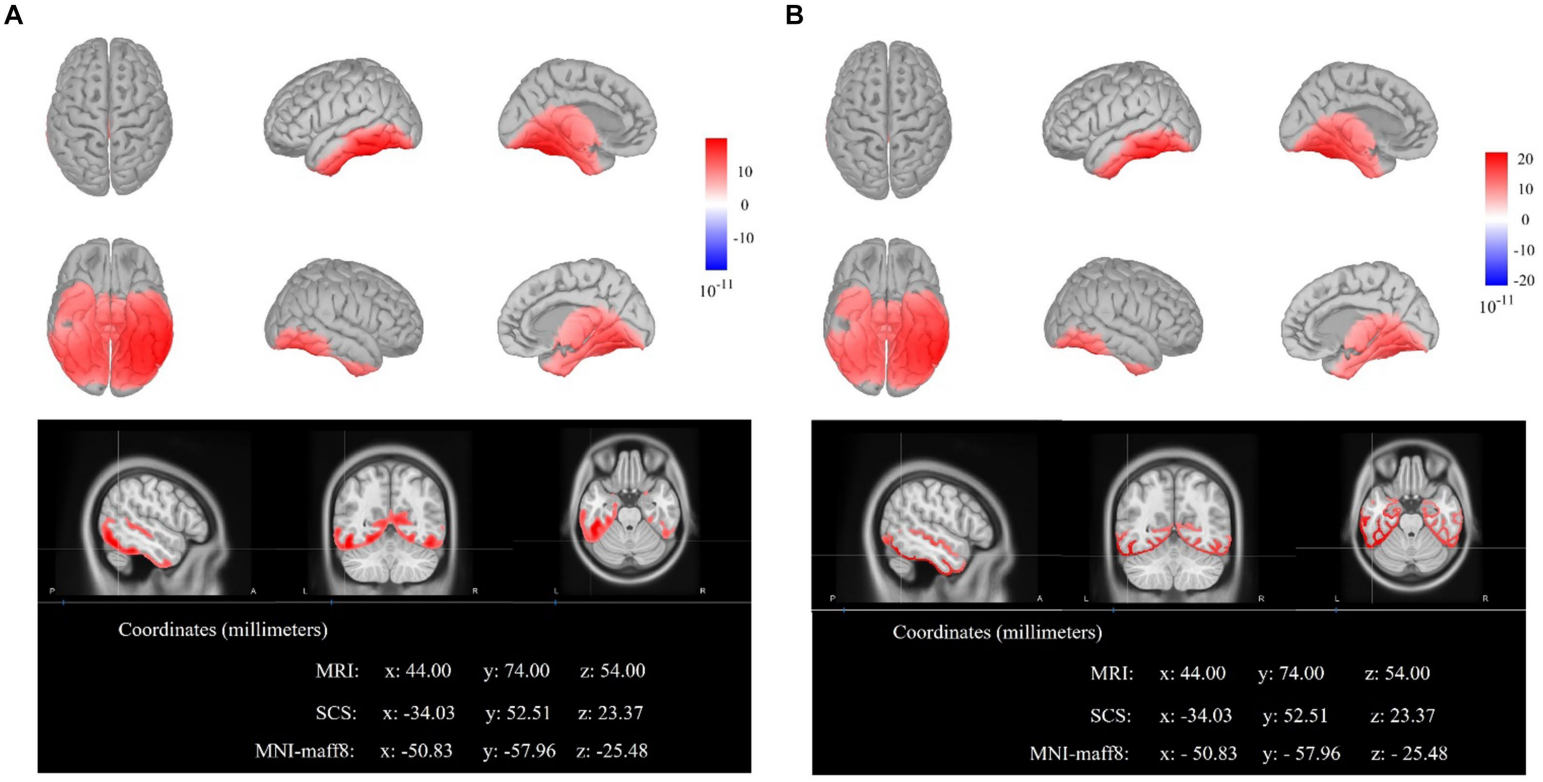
The electroencephalogram source locations of the difference waves (strong minus weak). (A) The sLORETA displays of activated neural source on cortex and MRI for the time window of 300–400 ms. (B) The sLORETA displays of activated neural source on cortex and MRI for the time window of 400–500 ms.
